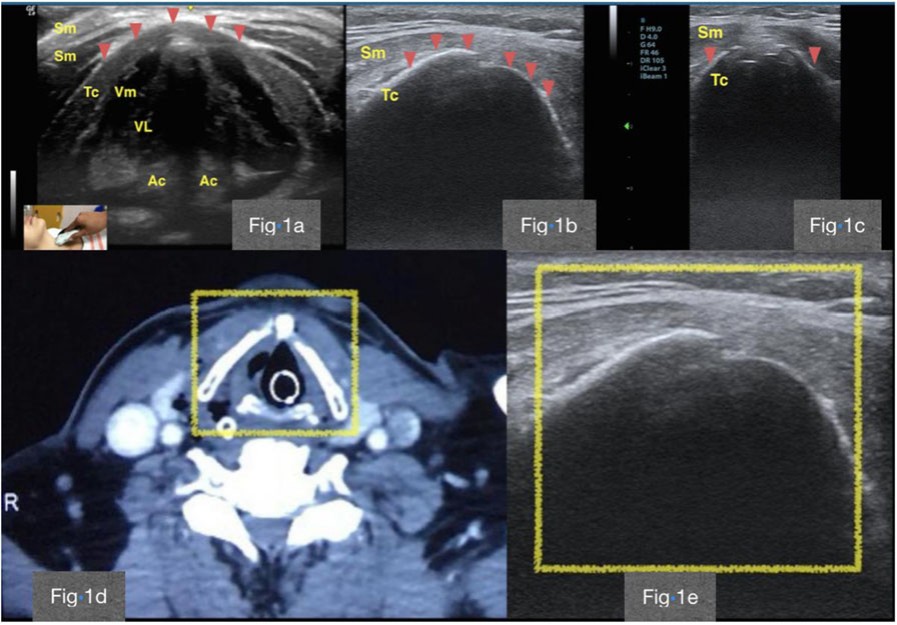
Figure 1a: Normal sonoanatomy in transverse scan. Figure 1b: Discontinuity of anterior cortex of thyroid cartilage with surrounding tissue oedema in transverse plane. Figure 1c: POCUS image of tranverse section of airway showing air mucosa disruption with a displaced thyroid cartilage. Sm-sternocleidomastoid muscle; Tc-thyroid cartilage; Vm-vocalis muscle; VL-vocalis ligament; AC- arytenoid cartilage. Figure 1d: A Computerised Tomography (CT) scan image shown defect in posterolateral wall of trachea with fracture of right anterior lamina of thyroid cartilage and superior cornu of left thyroid cartilage. Figure 1e: Figure of POCUS airway of thyroid cartilage in relation to the CT scan image
